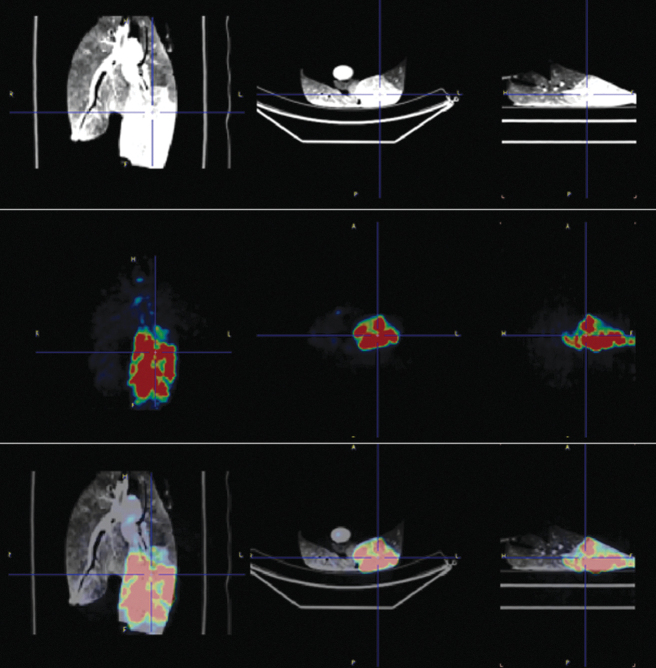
Imaging of left lower lobe delivery of 18F-FDG-labeled cells experiment. From the left to right: coronal, axial, and sagittal planes. Top row: CT imaging. Middle row: PET imaging. Bottom row: Fused CT and PET imaging showing the isotope-labeled cell distribution. 18F-FDG, fluorodeoxyglucose; PET, positron emission tomography.
